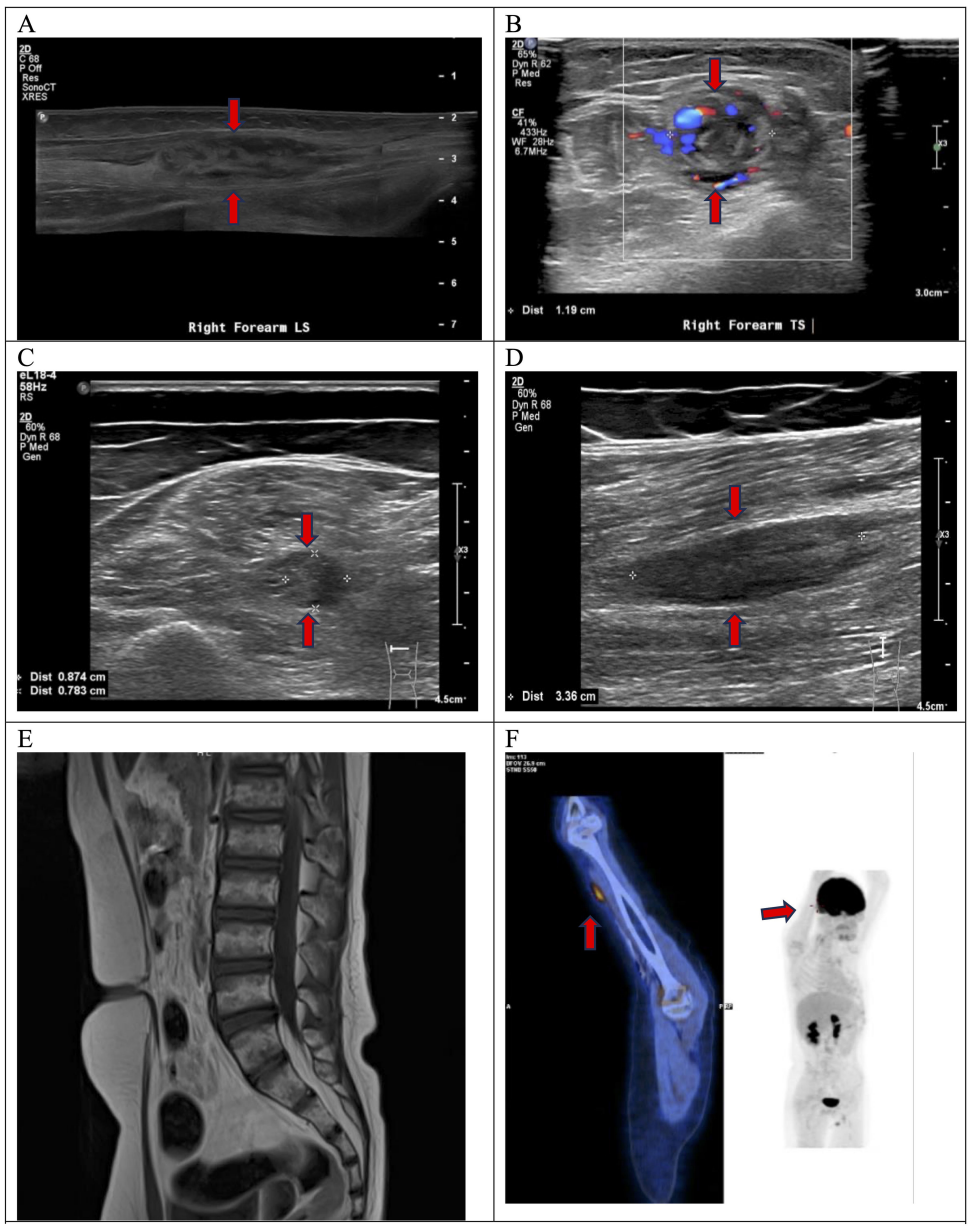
At the right mid-forearm, USG demonstrated an elongated heterogeneous lesion along the right ulnar nerve (A), measuring 1.3x1.2x5.8cm in size with increased intralesional color doppler signal seen on transverse view (B). At the left mid-thigh level, transverse view showed an abnormal fusiform swelling of medial component of the left sciatic nerve affecting the tibial trunk (C). Longitudinal view confirmed that the swelling was intraneural. It measured 0.9x0.8x3.4cm in size (D). MRI spine showed no compression of cauda equina (E). FDG PET-CT showed increased FDG avidity at anterior right mid-arm with SUV max 3.1 (F).
